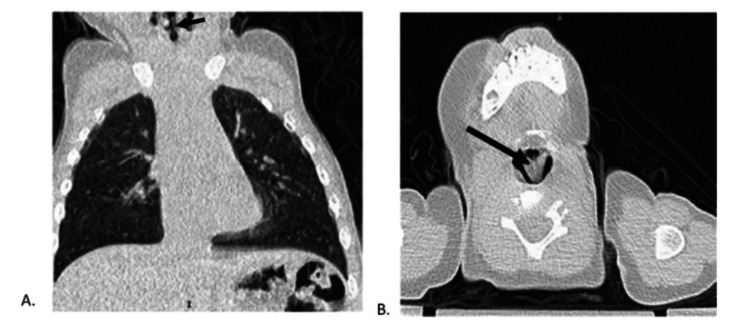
Computed tomography images of the chest A) Chest CT sagittal view demonstrating a thin foreign body in the glottis (arrow), consistent with a thin plastic sequin star B) CT neck axial view further demonstrating a thin plastic foreign body in the glottis.
